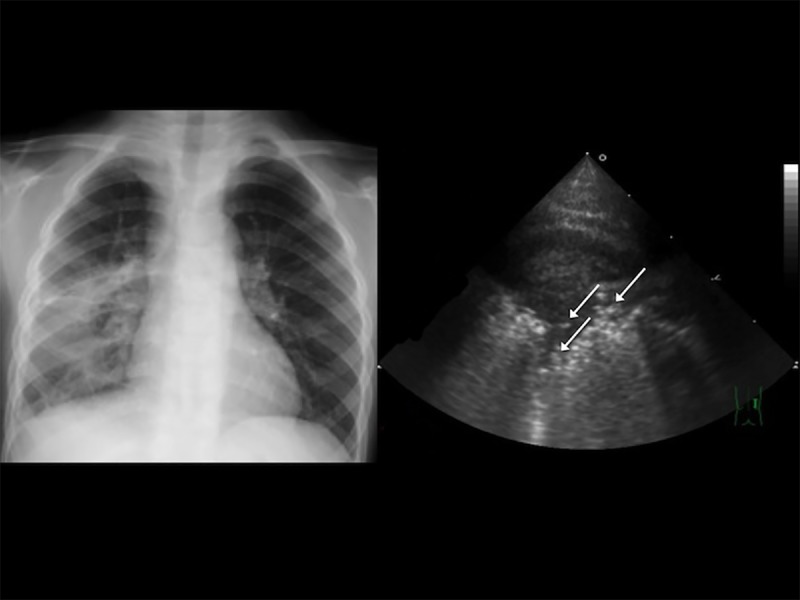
A 4-year-old boy with a high fever and dyspnea, classified as having severe pneumonia in our study. (A) Chest radiography revealed right lower lobe consolidation. (B) Transthoracic ultrasonography showed multiple hyperechoic spots in the right anterior lower lung, indicating the presence of air bronchograms (arrows).
